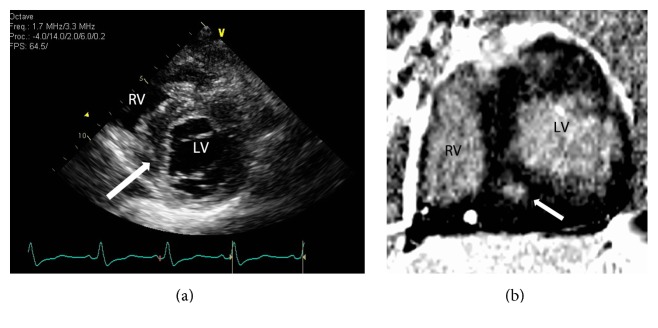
Sample short axis images from echocardiography (a) and cMRI (b) performed on a 12-year-old female patient with HCM demonstrating a* false negative* result on echocardiography. (a) demonstrates no corresponding focus of hyperechogenicity (white arrow) within the septal myocardium, which is apparent on cMRI (b) that confirms the presence of LGE within the same location indicating fibrosis. (RV: right ventricle and LV: left ventricle).
